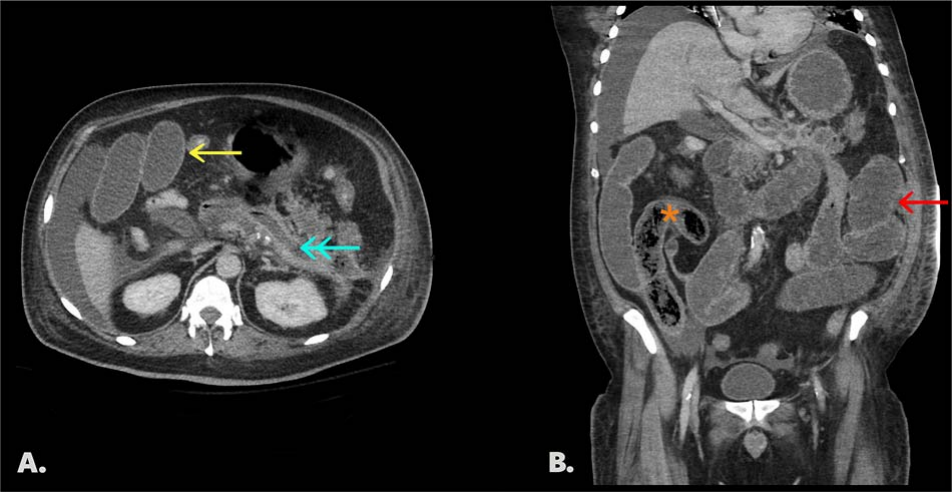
Follow-up CT with IV contrast (portal venous phase). Fig. 2A: an axial image shows a collapsed pancreatic bed (↞) containing trace fluid, dilated small bowel loops (←), and perihepatic free fluid. Fig. 2B: coronal image of same study shows interval migration of the bezoar into the distal small bowel (*) and upstream dilated, fluid-filled small bowel (←).
